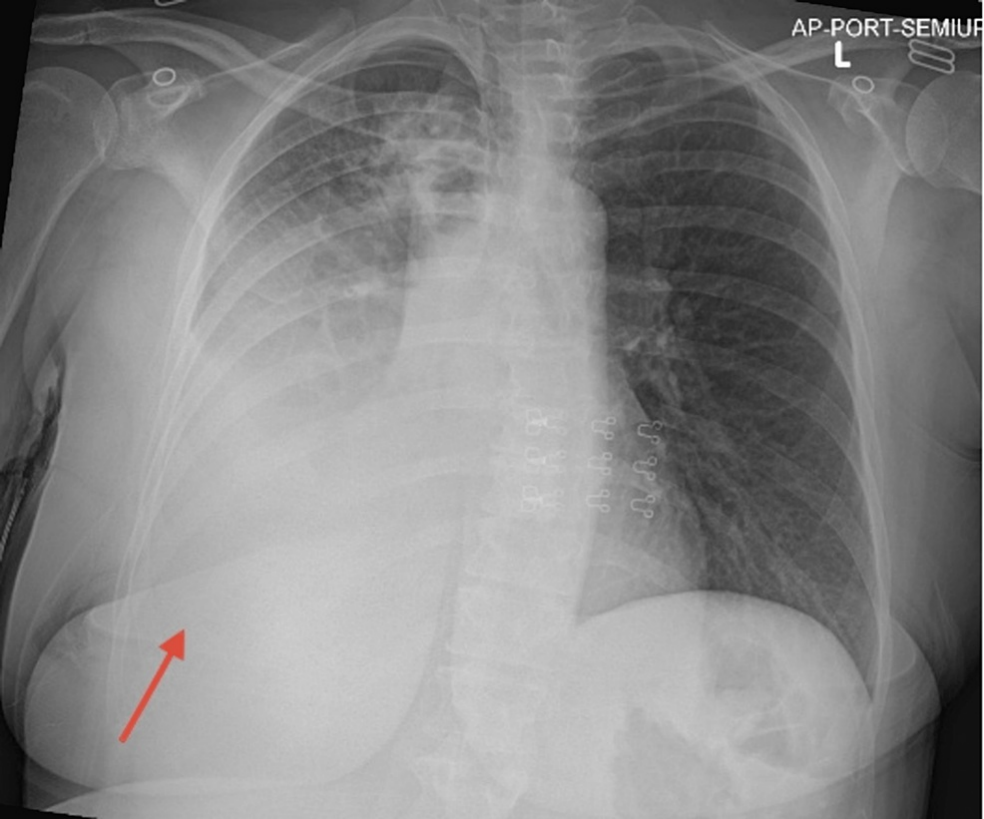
Initial Chest X-ray Upon Admission Red arrow indicating moderate to large right pleural effusion.
